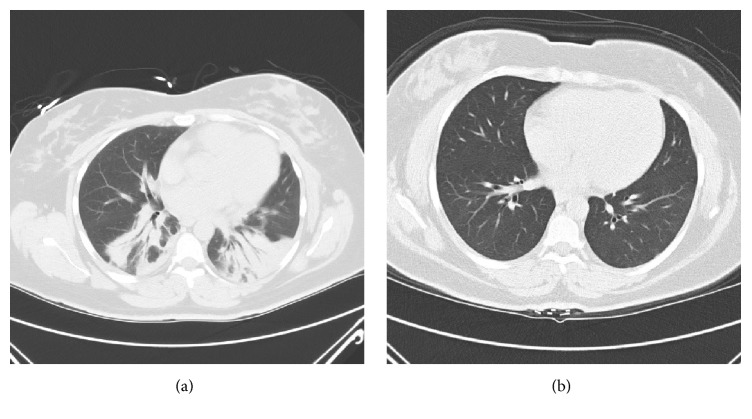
Computed tomography (CT) scans of case subject lungs prior to (a) and after (b) discontinuation of hydralazine therapy.
